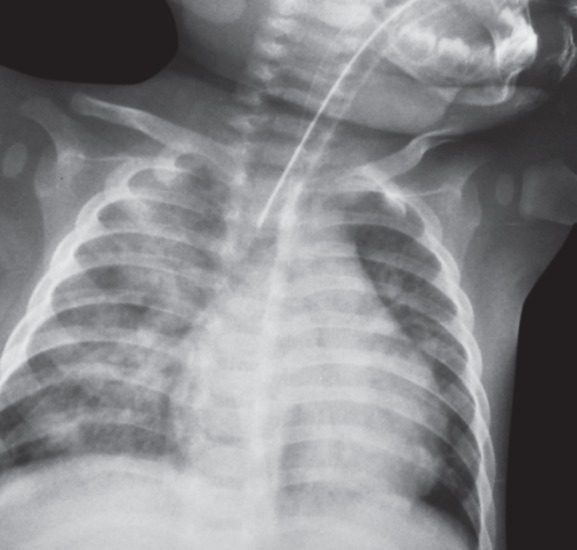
Chest X-ray of case 1 showing pulmonary hemorrhage
